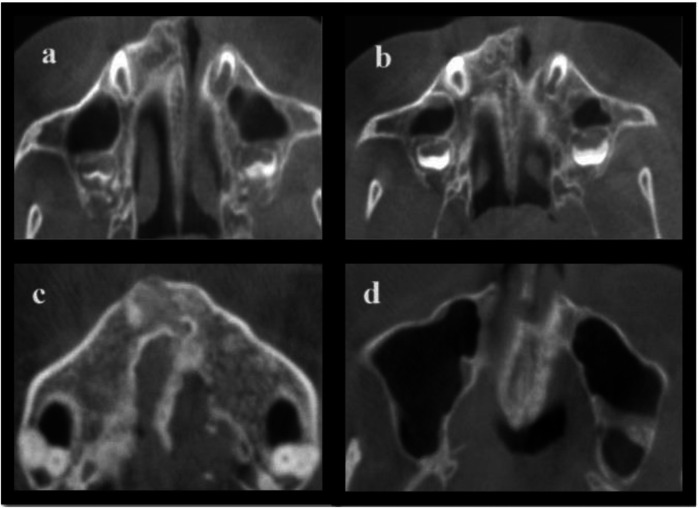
Represents the following stages of MPS in axial view of CBCT images: (a) A thick, visible, straight MPS line with minimal interdigitation; (b) A dense MPS line is seen that is irregular in shape; (c) Two scalloped, high-density MPS lines are seen; (d) The fusion of MPS is seen from posterior to anterior region of the palatine bone.
